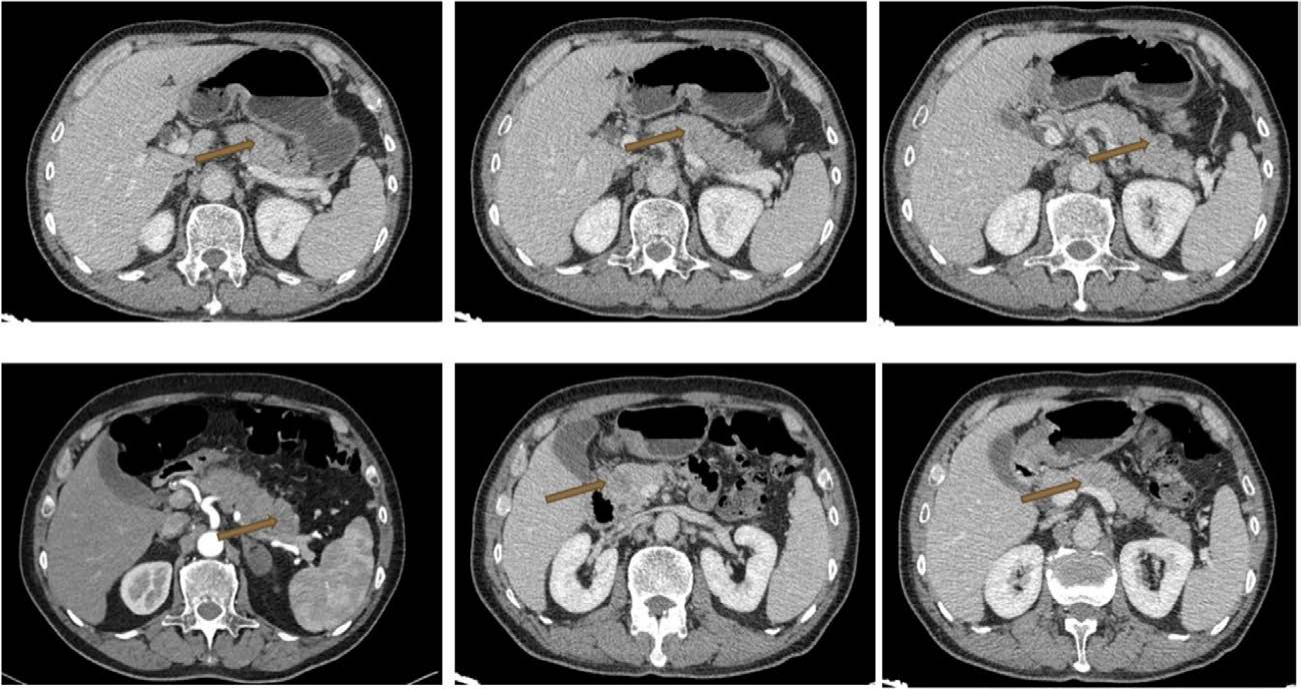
Abdominal enhanced CT at admission. CT = computer tomography.
